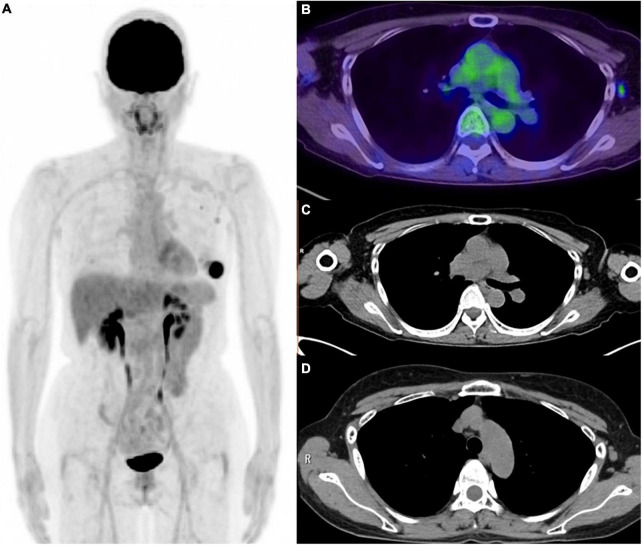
Six weeks after the COVID-19 vaccination for the patient with left breast cancer. (A) MIP image of FDG PET, (B) axial image of PET/CT, (C) CT portion of PET/CT, (D) CT image (2 weeks after the vaccination). Weak FDG uptakes were confirmed in the small axial lymph node and subclavian lymph node. These lymph node was pathologically diagnosed as lymph node metastasis from left breast cancer. Axial lymph node in CT image obtained 2 weeks after the vaccination was slightly larger than those in the PET/CT image, which might be the effect of vaccination.
